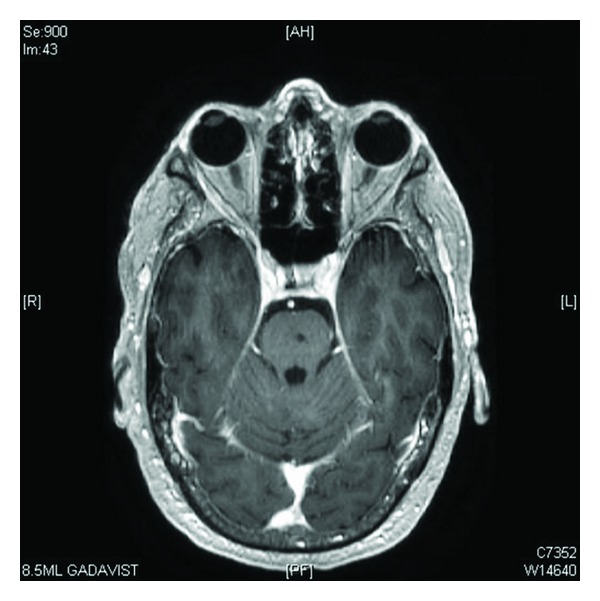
Posttreatment axial MRI image of the pons with complete ablation of the pontine metastasis and no further visible disease within the brainstem.
